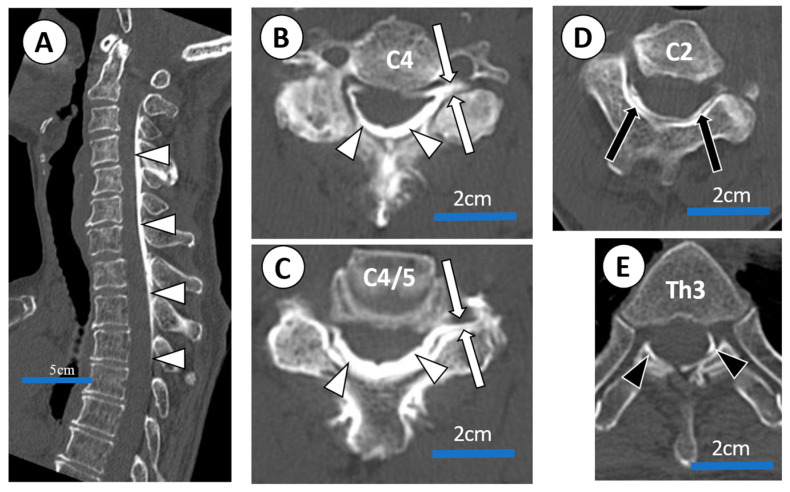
CT epidurogram after C4/5 US-guided epidural injection administered to a 73-year-old female with severe posterior cervical pain and right shoulder pain (case 4) associated with cervical canal stenosis. (A) Sagittal view of CT epidurogram. Dorsal spread (white arrow heads) between C2 and Th3 are confirmed. (B,C) Axial view of CT epidurogram (a slice of C4 vertebra and C4/5 disc; injection site). Dorsal spread (white arrow heads) and C5 root filling (white arrows) were confirmed. (D,E) Axial view of CT epidurogram at C2 and Th3 vertebra ). Cephalad spread (black arrows) was confirmed up to C2 vertebra level and caudal spread (black arrow heads) was confirmed down to Th3 vertebra level.
